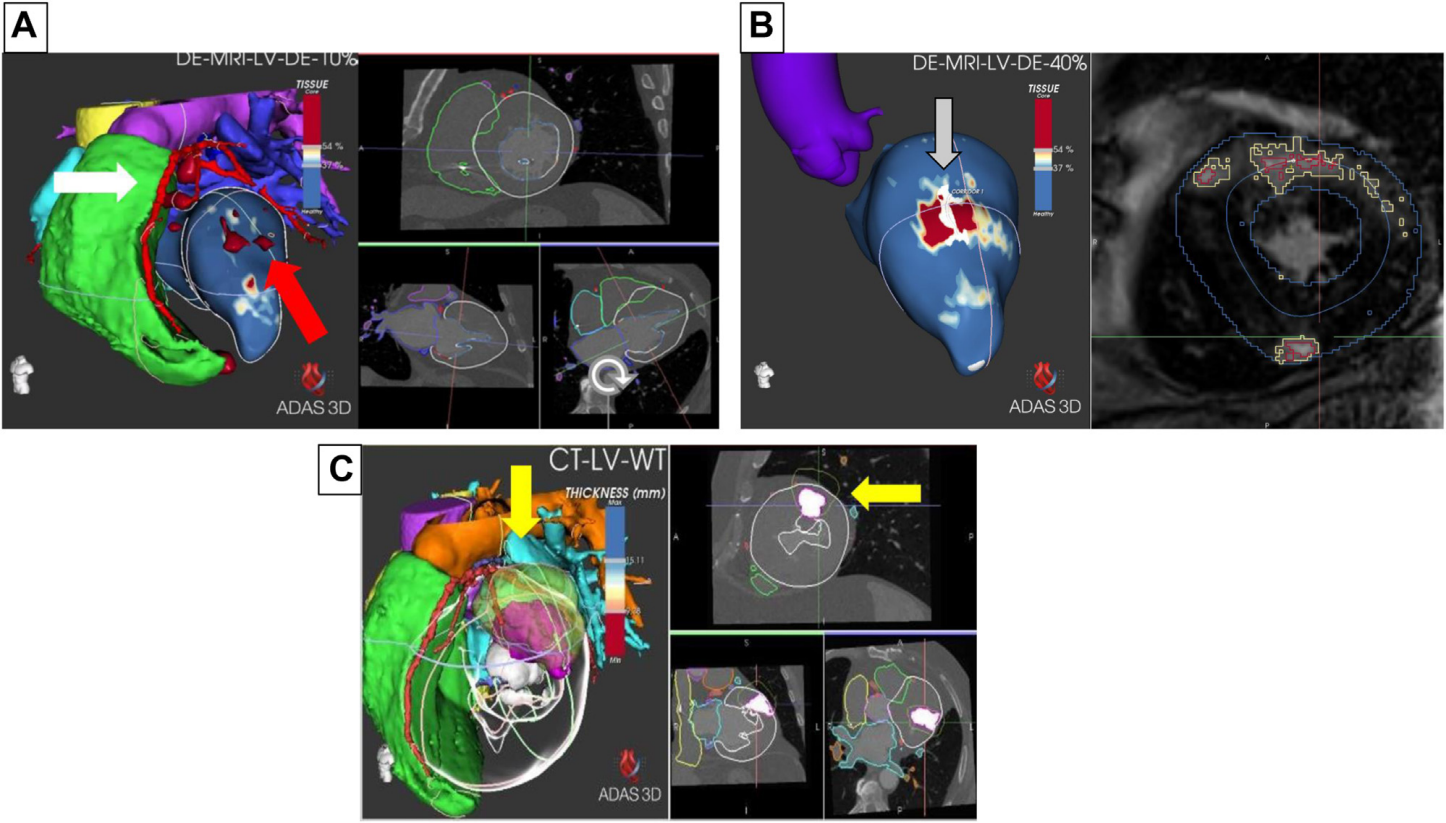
ADAS 3D (Barcelona, Spain) reconstruction. A: Cardiac computed tomography (CT) and magnetic resonance imaging (MRI) fusion. The left ventricle is represented in layers corresponding to myocardial depth and this image belongs to the endocardium (10%). Cardiac structures are represented as follows: right ventricle (*green*), pulmonary artery (*purple*), aorta (*yellow*), left atrium (*blue*), and coronary arteries (*white arrow*). Red arrow points out intramyocardial substrate observed in MRI. B: MRI reconstruction with ADAS shows intramyocardial layer (40%). Grey arrow points out an intramyocardial corridor, corresponding to ventricular tachycardia critical isthmus. C: Cardiac CT / electroanatomical map and radiotherapy planning CT fusion. Area of interest delimited in electroanatomical map is represented (*yellow arrow*).
